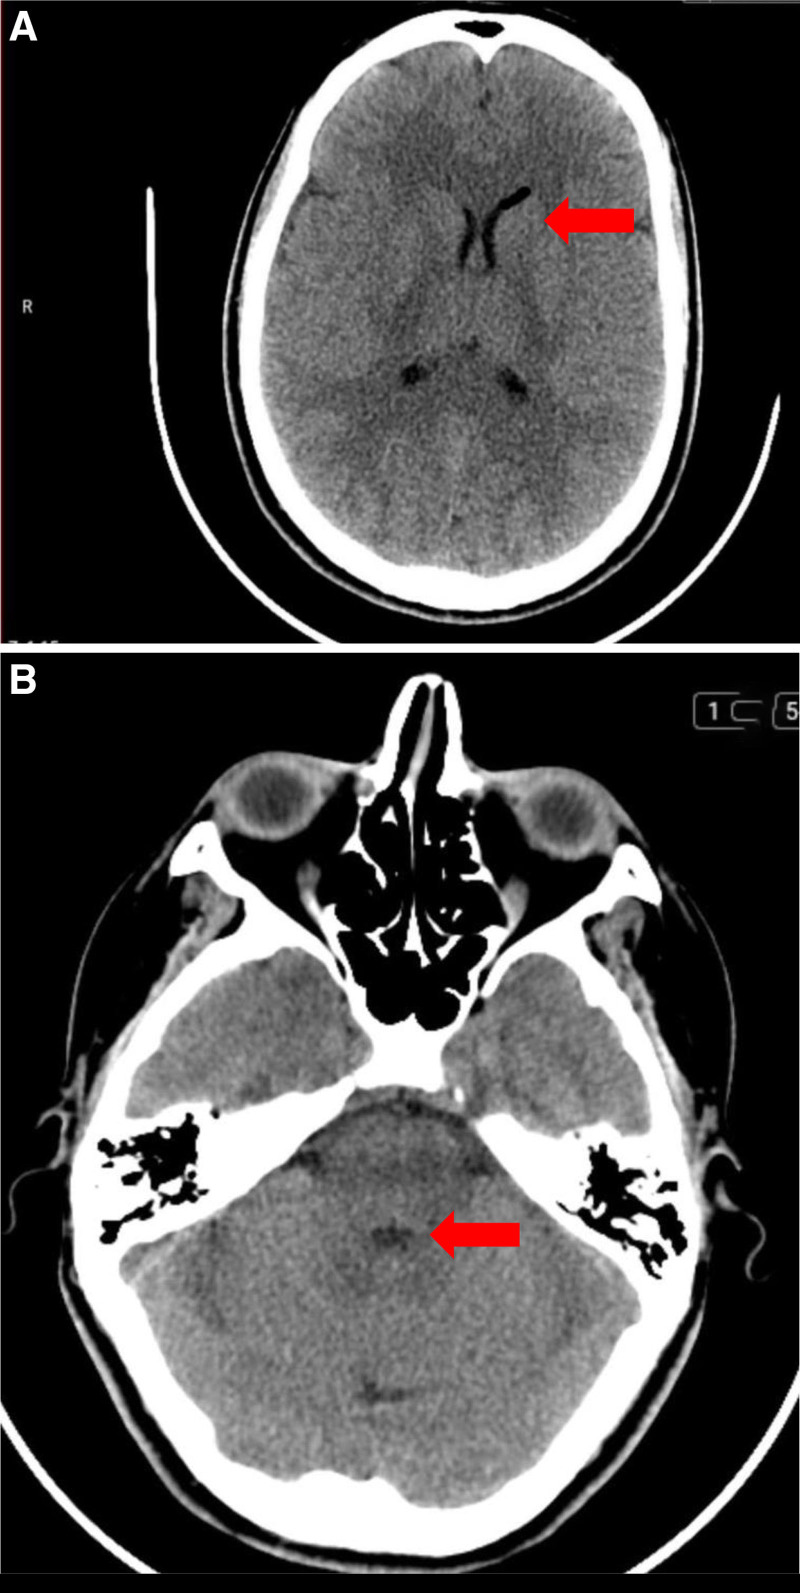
(A) Follow-up axial non-contrast CT scan of the brain demonstrating partial resolution of intracranial air (red arrow), with normalization of ventricular anatomy and with minimal residual pneumocephalus. (B) Follow-up axial non-contrast CT scan showing complete resolution of the previously seen peripontine pneumocephalus, with no residual intracranial air (red arrow). CT = computed tomography.
